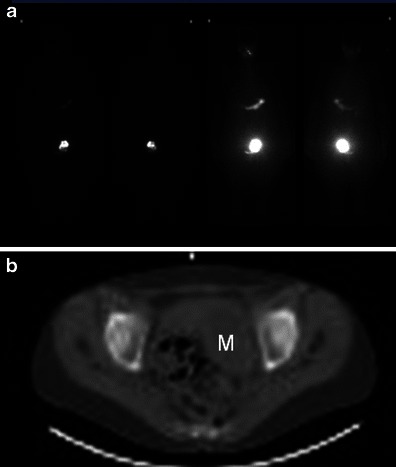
a Whole-body I-123 imaging shows significantly increased I-123 uptake within the left hemi-pelvis in a 61-year-old woman with a history of thyrotoxicosis treated with radioiodine treatment twice over the last 30 years, who ultimately had a thyroidectomy which showed Graves disease but who remained thyrotoxic post surgery. b The area of increased uptake within the left hemi pelvis corresponds on the low-dose CT to an 8-cm left-sided pelvic mass (*M*) in keeping with a confirmed struma ovarii
